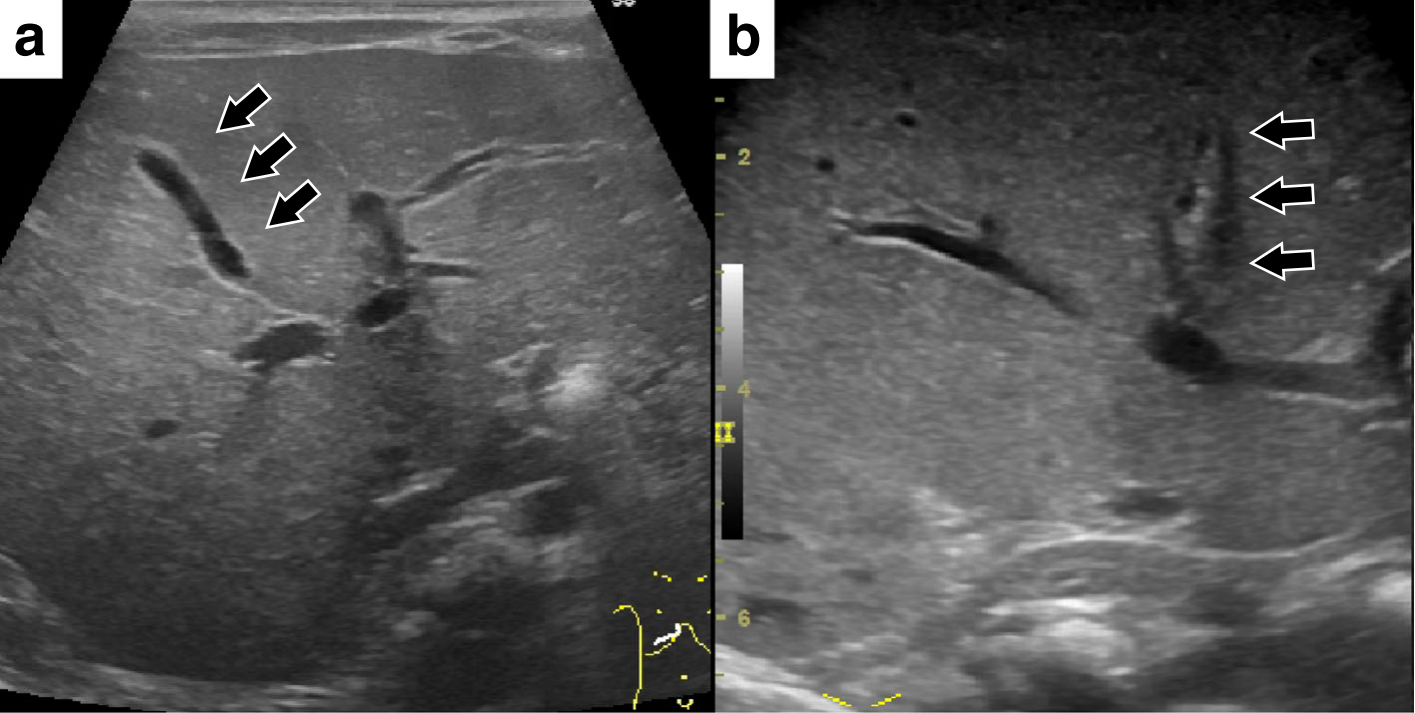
Preoperative abdominal ultrasonography. a Six hours after oral feeding. A gallbladder with a diameter of 19 mm was isolated. b The gallbladder contracted 1.5 h after oral feeding
